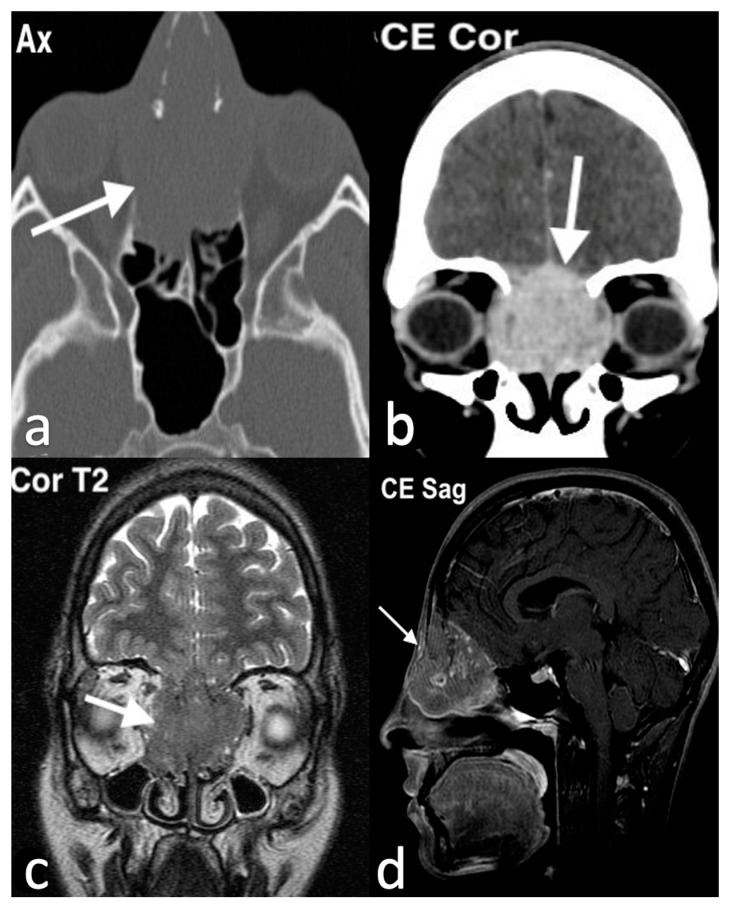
Esthesioneuroblastoma in a 22-year-old girl with headache worsening in the supine position, associated with serous rhinorrhea and anosmia. She also had pain at the root of the nasal pyramid (widened and deformed) and eyelid edema. Endoscopically, at the level of the olfactory slit, an oval mass with a smooth surface and yellowish color, covered by a dense vascular reticule, was bilaterally evident. On the axial CT image (a), a mass (arrow) in both nasal cavities, with marked erosion of ethmoid and nasal bones, is visible; on the contrast-enhanced coronal CT image (b), the mass (arrow) shows intense and inhomogeneous enhancement, with massive erosion of ethmoid, involving the medial wall of the orbits and cribriform plate. MRI: on the coronal T2 image (c), the mass (arrow) shows low–intermediate intensity, with invasion of extraconic orbital fat; transcompartmental extension is also visible with intracranial extension in the olfactory groove; on the sagittal fat saturation T1 after intravenous Gd image (d), the tumor (arrow) shows heterogenous enhancement.
